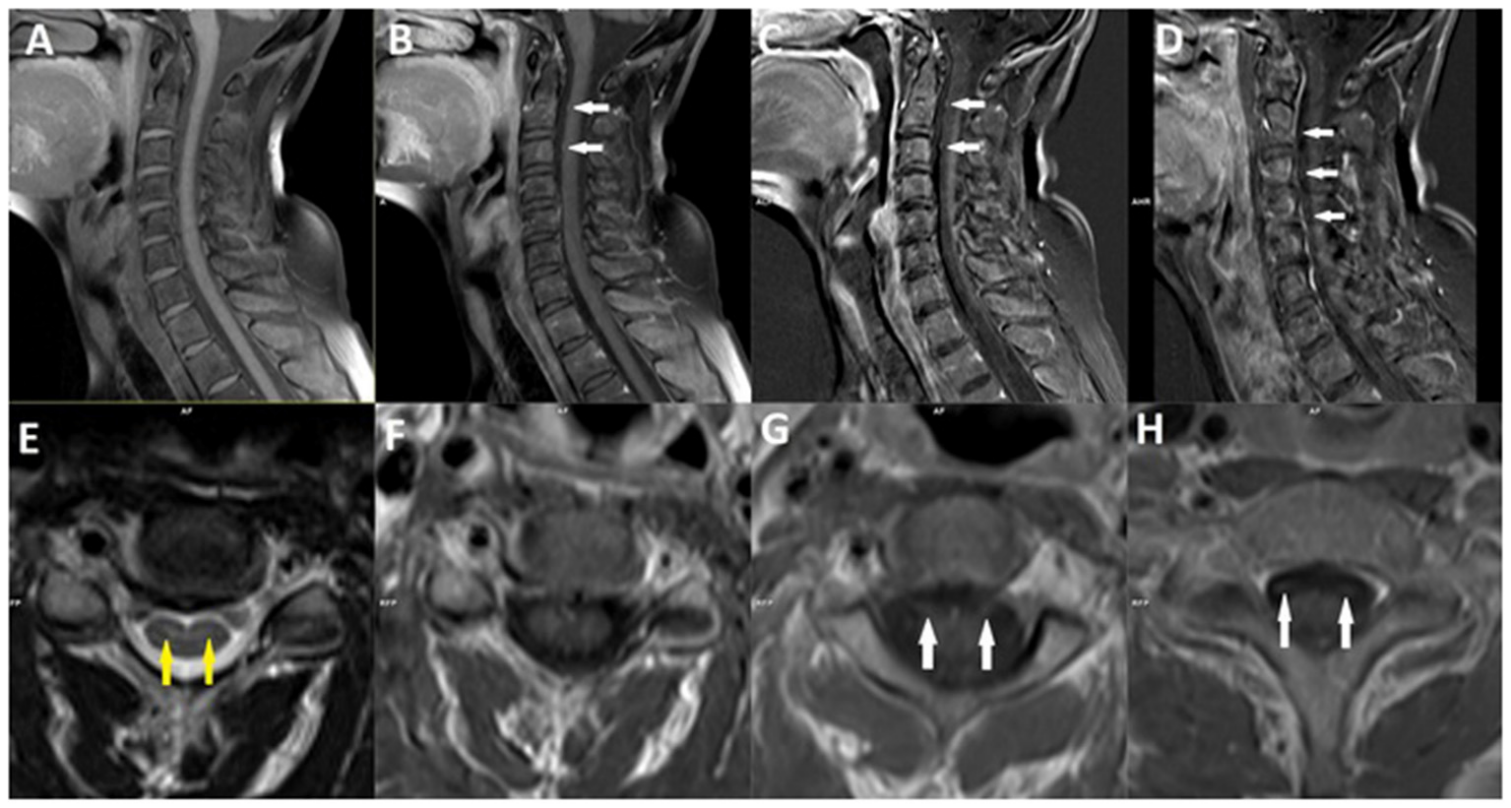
Magnetic resonance imaging of the cervical spine of a patient with chronic inflammatory demyelinating polyneuropathy (CIDP) after COVID-19 infection. Sagittal (A) and axial (F) precontrast T1-weighted images, and postcontrast sagittal (B) and axial (G, H) T1-weighted images with sagittal T1-weighted subtraction maps (C, D), demonstrate bilateral enhancement of the ventral nerve roots (*white arrows*). Axial T2-weighted image (E) demonstrate discrete the hyperintensities in the anterior horns of gray matter (*yellow arrows*).
